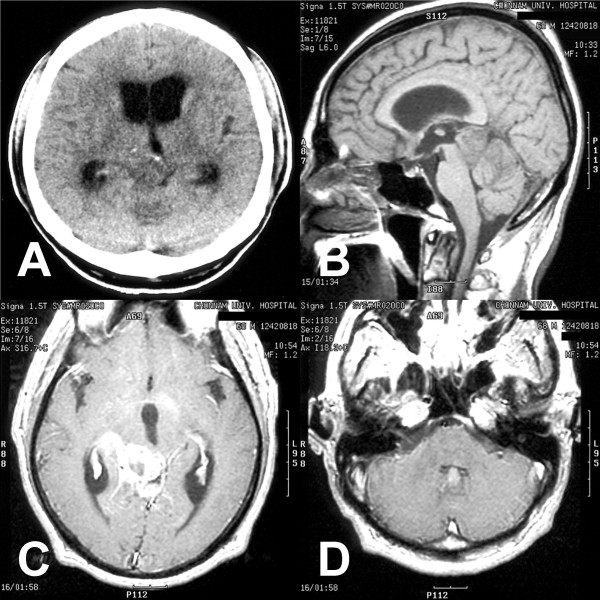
Non-contrast computed tomography scan showing a hypointense mass in the pineal region (A). T_1_-weighted sagittal (B) and gadolinium-diethylenetriaminepentaacetic acid enhanced axial (C and D) magnetic resonance images demonstrating a heterogeneously ring-enhanced mass with central necrosis in the pineal region and ependymal dissemination in the fourth ventricle.
